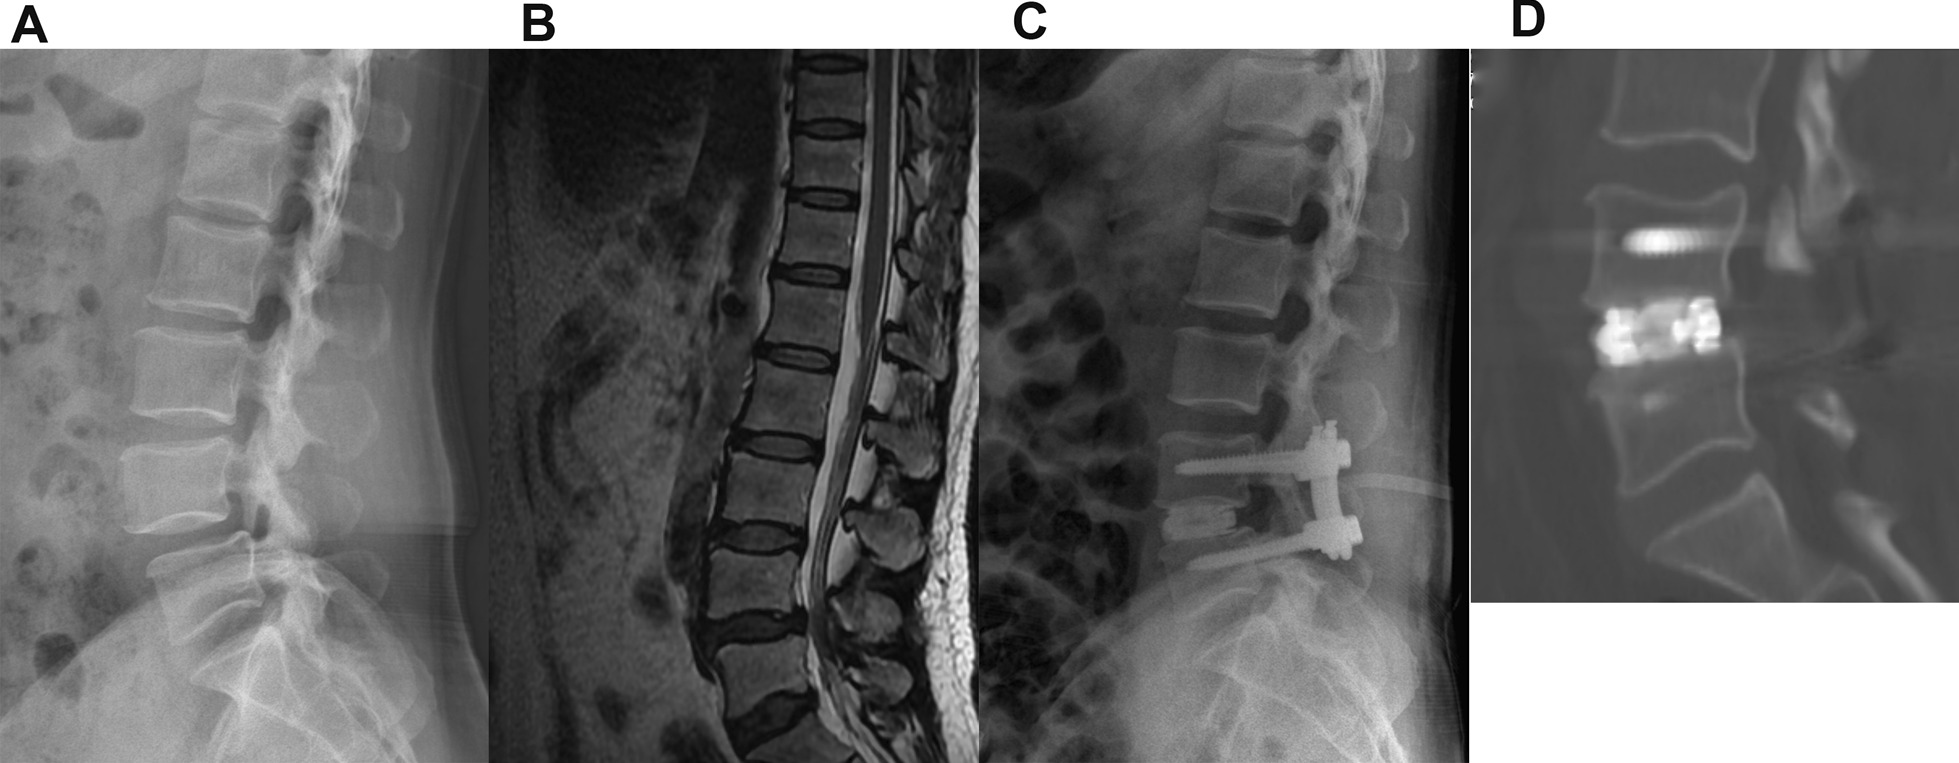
A 54-year-old male with L4-5 spinal canal stenosis, who was treated with percutaneous endoscopic transforaminal lumbar interbody fusion and percutaneous pedicle screw fixation. A Preoperative lateral radiograph (A) and MRI (B) show degenerative spondylolisthesis and canal stenosis with narrowed disc space at L4-5. C Postoperative lateral radiograph shows excellent screws and cage location with improved disc space height. D Sagittal CT obtained at the last follow-up duration (15 months after operation) shows interbody bony fusion
